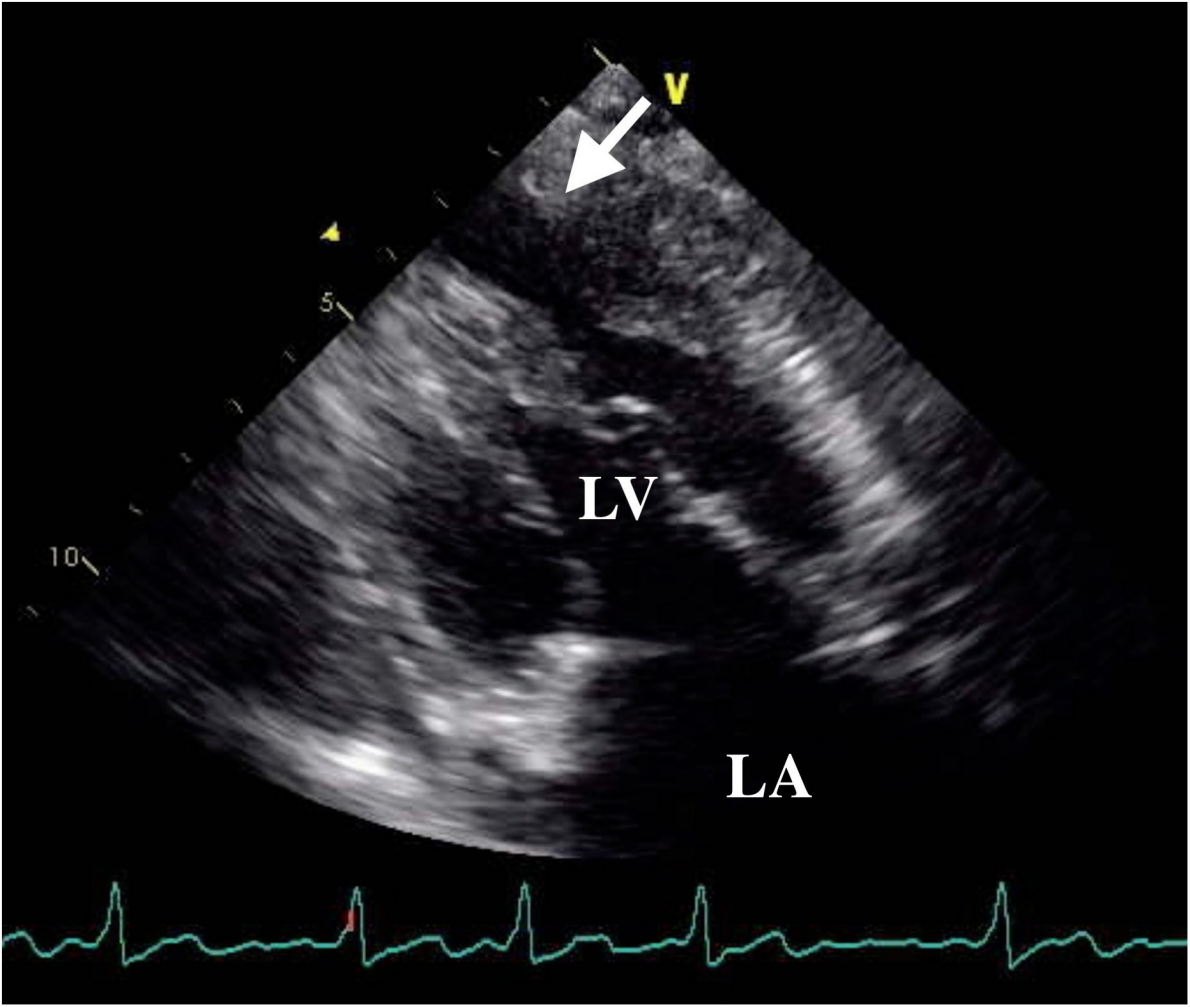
Transthoracic echocardiography imaging showing apical hypertrophic cardiomyopathy with left ventricular apical aneurysm (arrow). LA, left atrium; LV, left ventricular.
